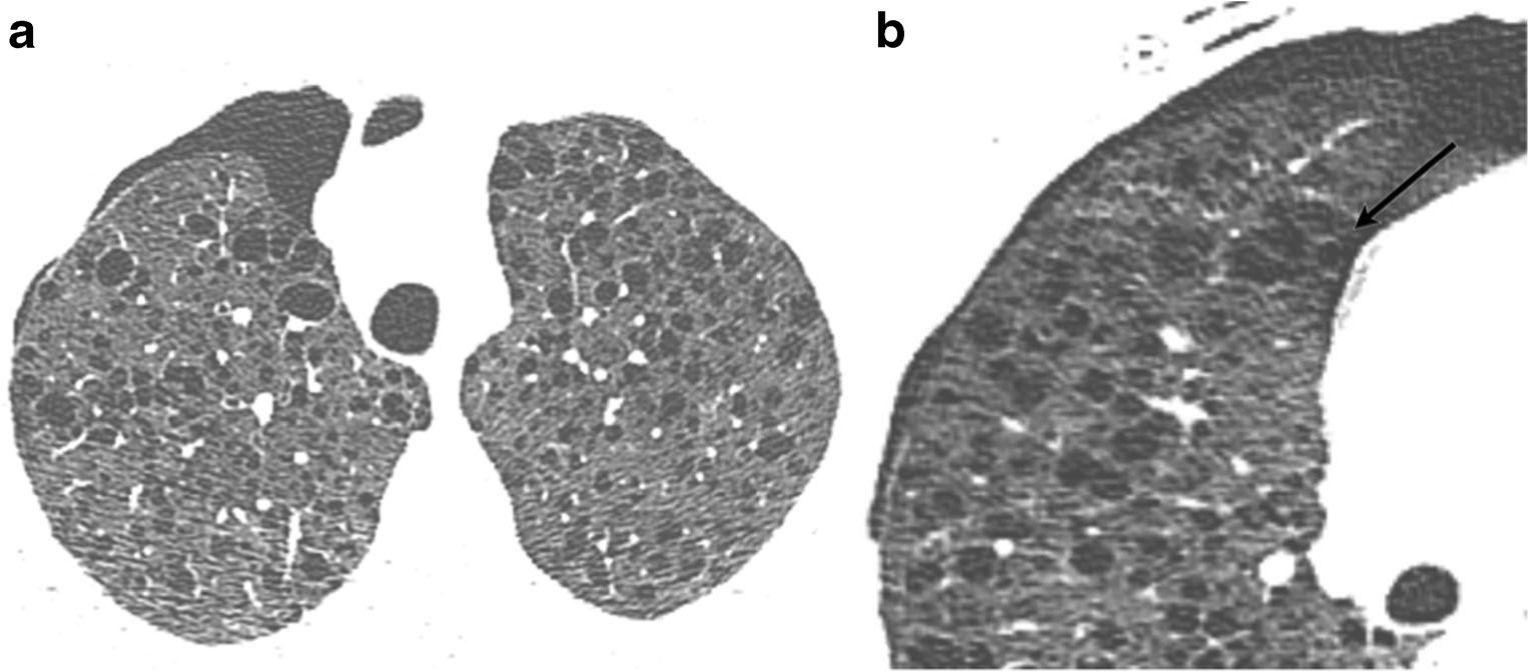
A 28-year-old patient with lymphangioleiomyomatosis, recurrent pneumothoraces and chest pain. Chest CT axial image (a) demonstrates multiple cysts in both lungs. Thin slice image (b) with a sharpened reconstruction kernel clearly demonstrates the discontinuity of the walls of a cyst (*arrow*) compatible with an alveolopleural fistula
